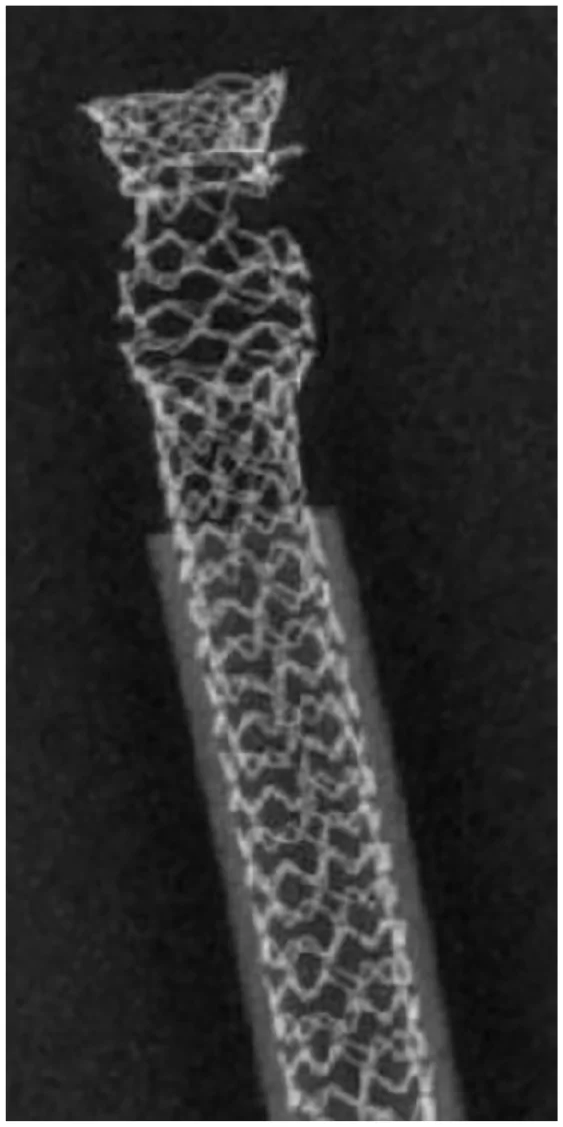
The digital radiography of a BeGraft stent used in a custom-made fenestration shows a partial fracture of the metallic frame after 40 million cycles.
